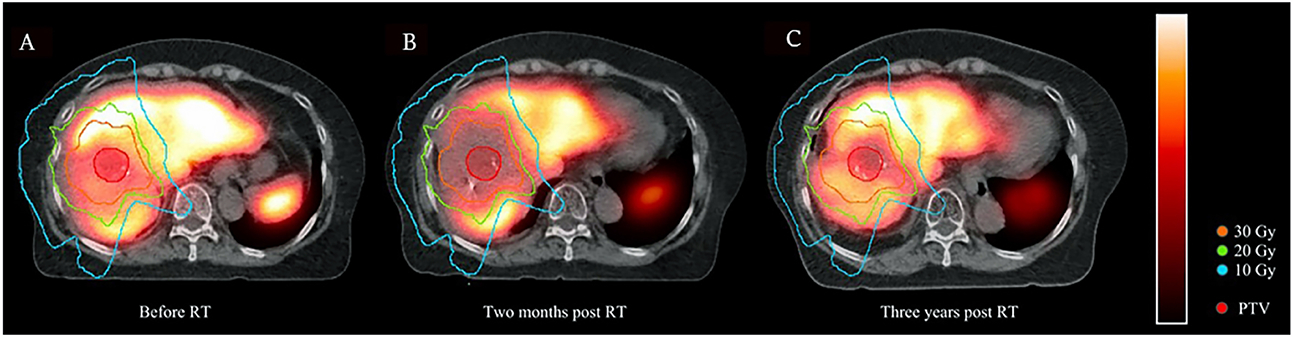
Functional liver recovery as imaged by serial longitudinal SC SPECT/CT in a CP-A patient who received multiple prior liver-directed therapies (chemoembolization, radiofrequency ablation, ethanol injection). (A) Radiation treatment isodose lines overlaid with pre-treatment SC SPECT/CT scan; (B) 2 months post-RT showing functional liver loss at 20 Gy and above; (C) 3 years post-RT showing functional liver recovery in regions that received 10-30 Gy. SC: Sulfur colloid; CP: child-pugh; RT: radiotherapy.
